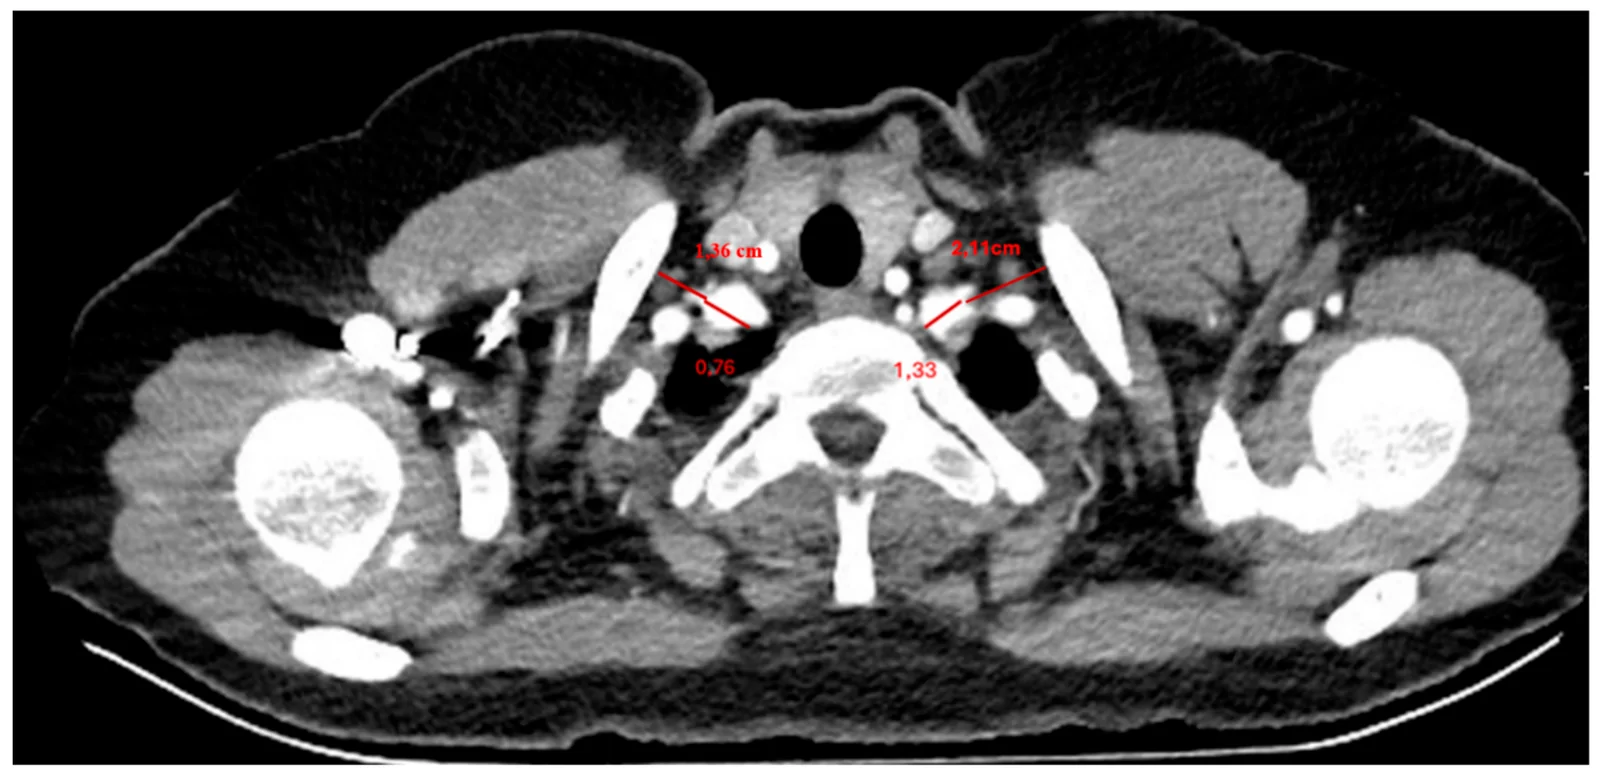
CTA-based visualization of the right internal thoracic artery origin. Coronal and sagittal oblique CPR images demonstrate the origin of the ITA from the subclavian artery and the measurement approach.
